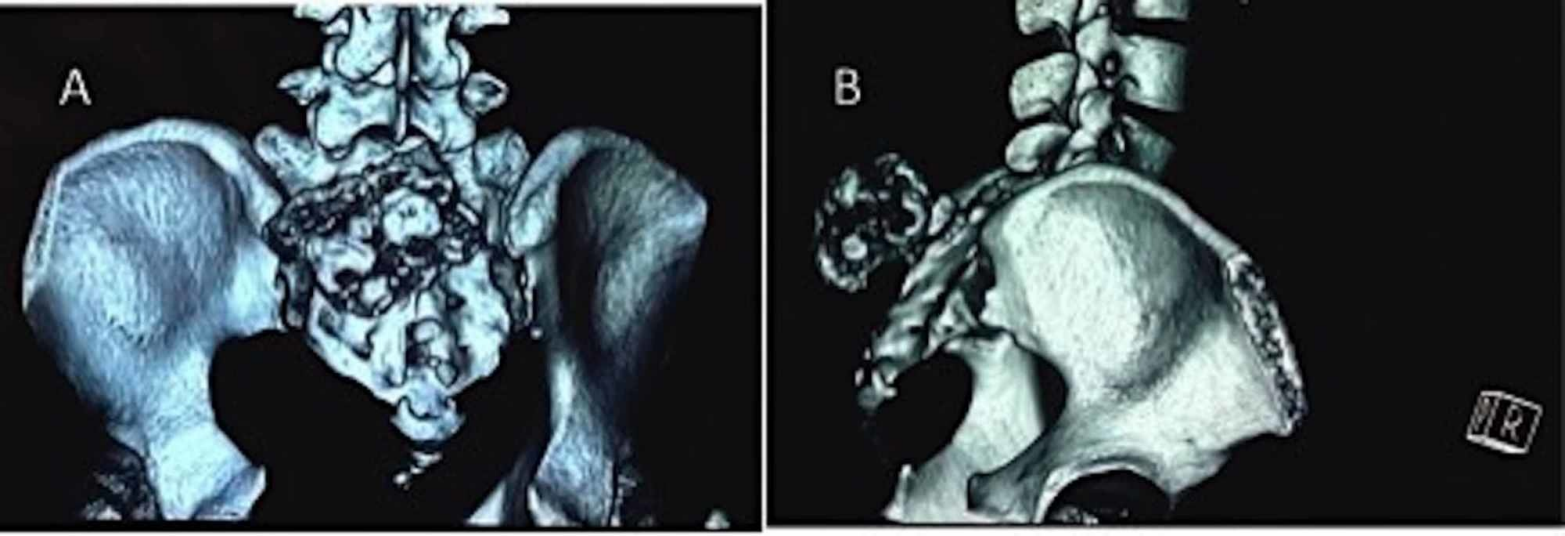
CT scan clearly delineates the growth on the left side of the sacrum arising from the S2-3 level
